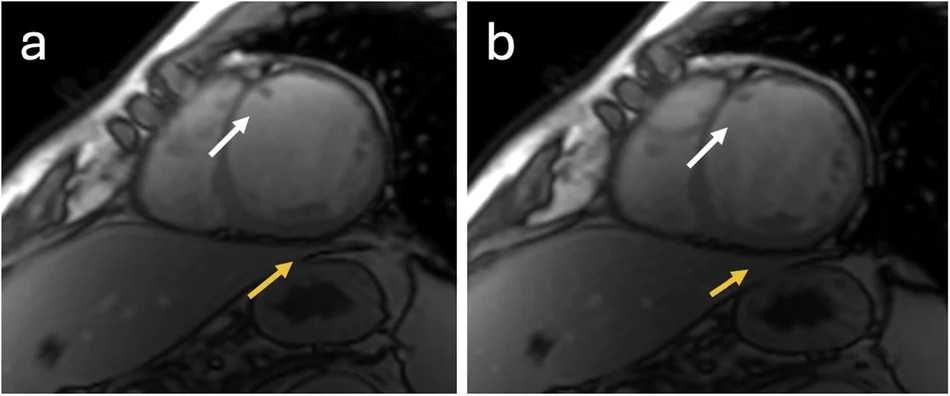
Inhomogenous signal intensity due to B1 inhomogeneity can be observed qualitatively by looking at the differences in signal intensity. The anterior region notably has higher signal intensity (white arrows), as compared to the inferior region (yellow arrows). Both images are taken from the 3-T MRI of the same patient but at different RR intervals: a 1050 ms and b 955 ms
